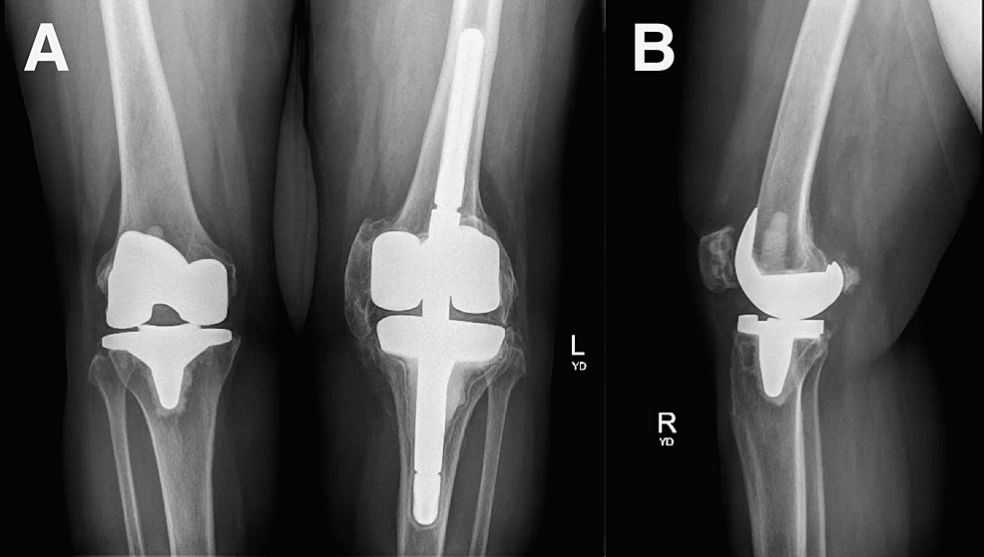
One-year postoperative bilateral anteroposterior (A) and right lateral (B) knee radiographs demonstrating no evidence of heterotopic ossification in the right knee.
